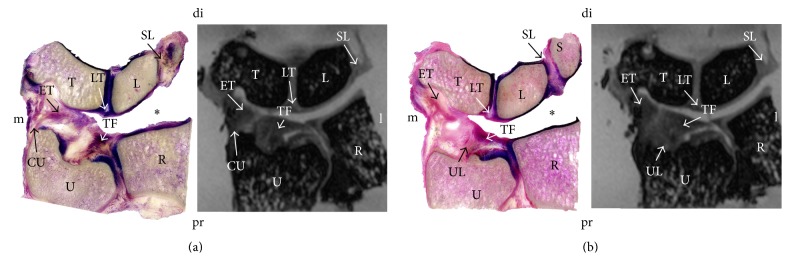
Plastination of the triangular fibrocartilage disc complex. Dorsal coronal section (a) and ventral coronal section (b) in a similar plane and orientation for plastination (left) and magnetic resonance imaging (right). Note the fibrocartilaginous attachments of the complex at the radius, ulna, and triquetrum. The asterisk (*∗*) indicates a deformation artifact introduced due to dehydration-related deformation. CU = collateral ulnar ligament; DRU = dorsal radioulnar ligament; ET = extension of the triangular fibrocartilage; LT = lunotriquetral ligament; SL = scapholunate ligament; TD = triangular disc; TF = triangular fibrocartilage; UL = ulnolunate ligament; L = lunate; R = radius; S = scaphoid; T = triquetrum; U = ulna; di = distal; l = lateral; m = medial; pr = proximal.
